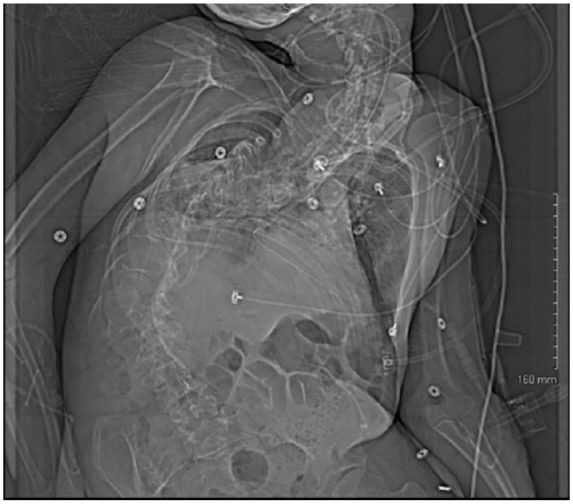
Scout chest X-ray showing severe kyphoscoliosis of patient with Coffin-Lowry
